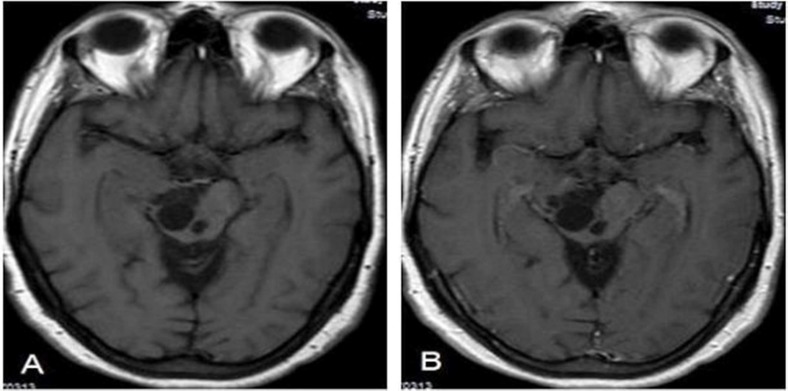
Axial T1-weighted (W) (A) and axial contrast-enhanced T1-W (B) magnetic resonance images reveal that there is no pathological contrast enhancement
